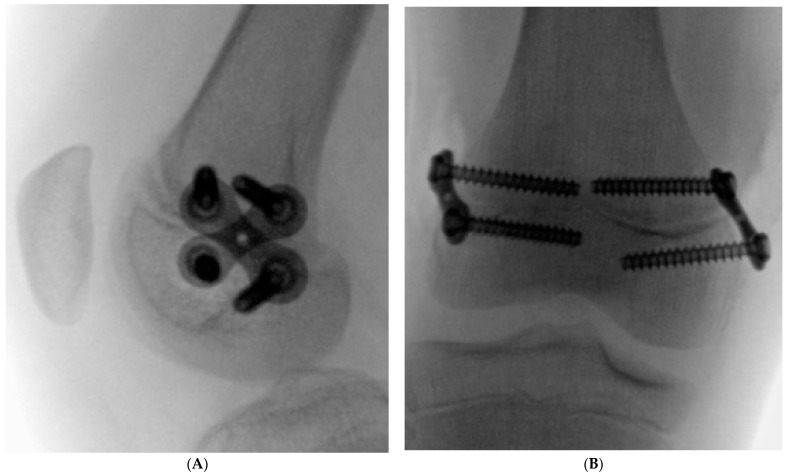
Intraoperative radiographs of an 8-plate positioned obliquely for RGG, with (A) showing the lateral view and (B) showing the anteroposterior (AP) view.
